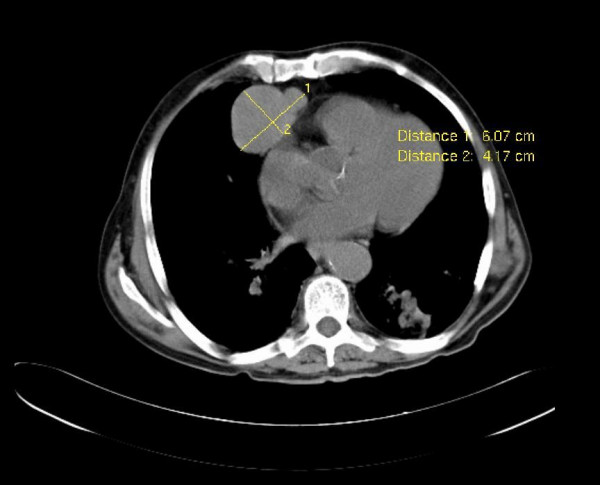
CT scan; CT scan confirmed mediastinal abnormality, demonstrating a 6-cm mass in the anterior mediastinum.
